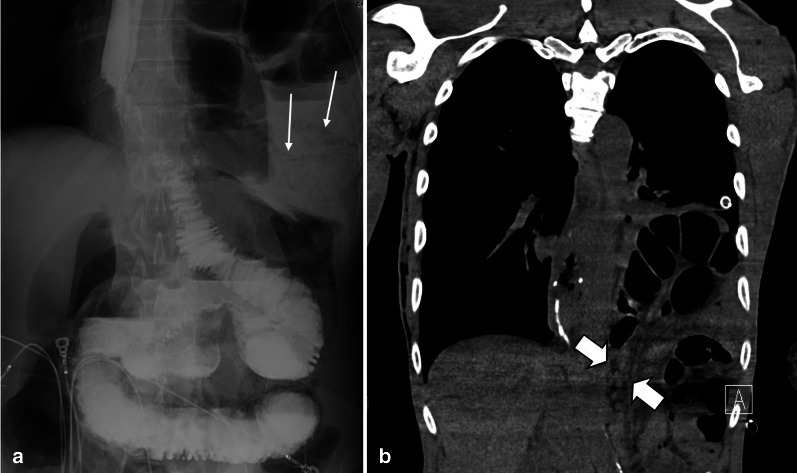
A 59 y/o male status post recent bowel resection for malignancy found to have leukocytosis and abdominal pain. a Postoperative left diaphragmatic hernia with pneumatosis (thin arrows) found during postop leak check fluoroscopic exam. b Follow-up CT confirmed a diaphragmatic defect (thick arrows) with herniation of bowel loops into the lower chest. Necrotic bowel was found at surgery
